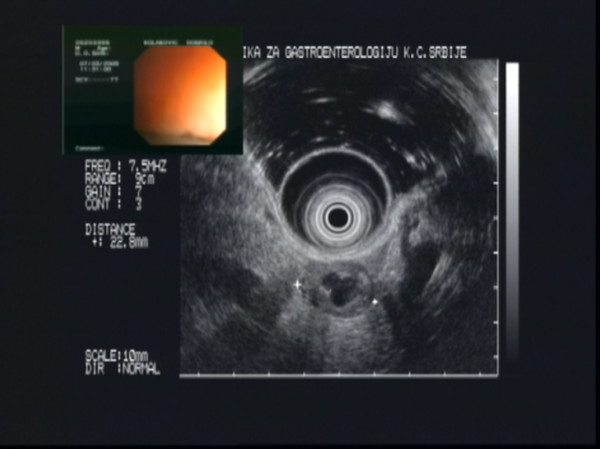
Endoscopic ultrasonography showing a cystic tumour in the pancreatic body (Olympus GIF-130 video echo-endoscope with 7.5/12 MHz switchable radial probe).
